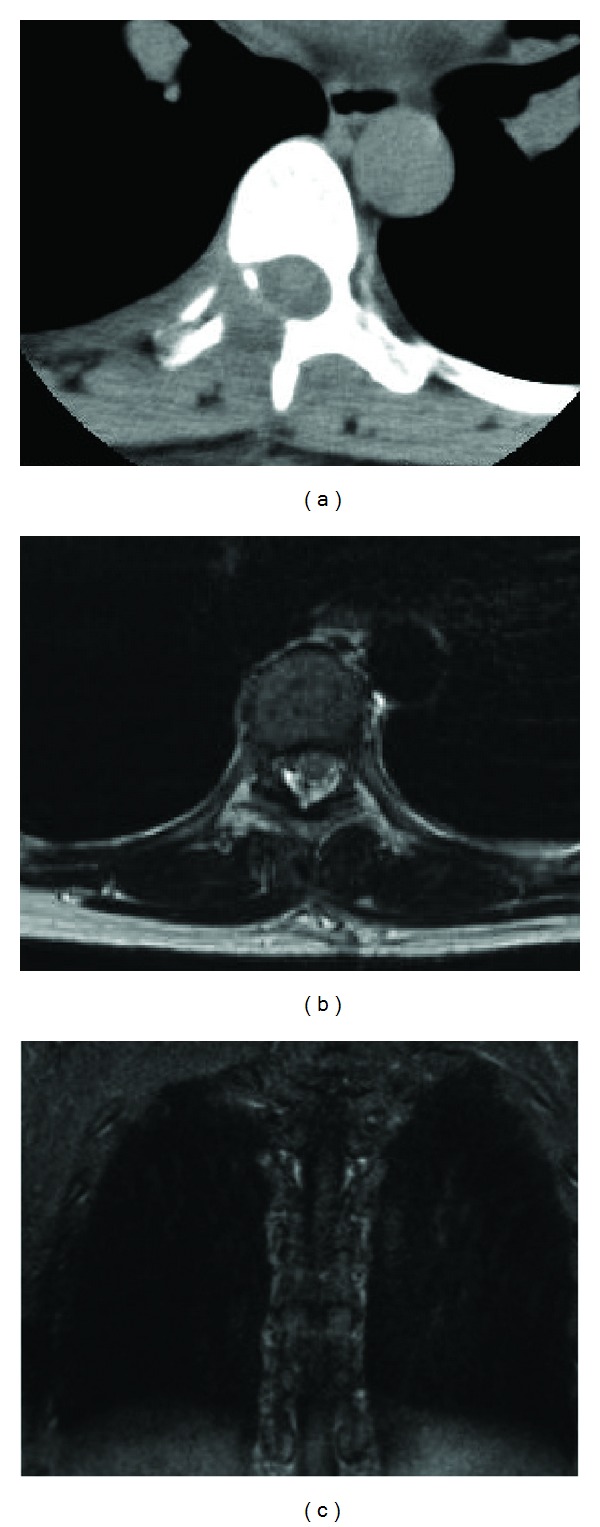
Postoperative hemilaminectomy of CT scan (a), axial (b), and coronal (c) views of T1-weighted MR images with gadolinium enhancement demonstrating complete removal of the tumor and decompression of the thoracic cord.
